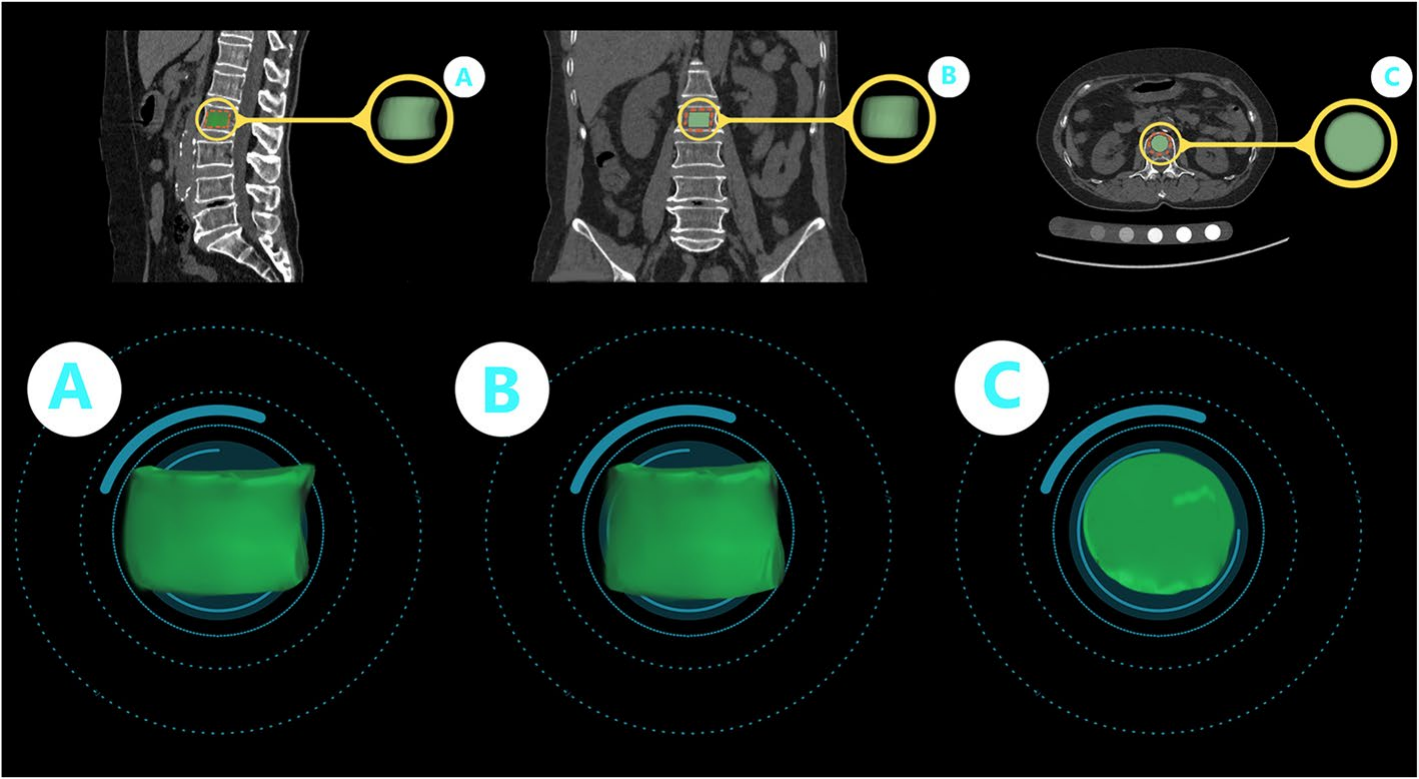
Representative volume-of-interest (VOI) in the 70-kev monochromatic image of the lumbar spine CT of an osteoporotic participant. A, B, C, VOIs on sagittal, coronal and axial CT images, respectively
